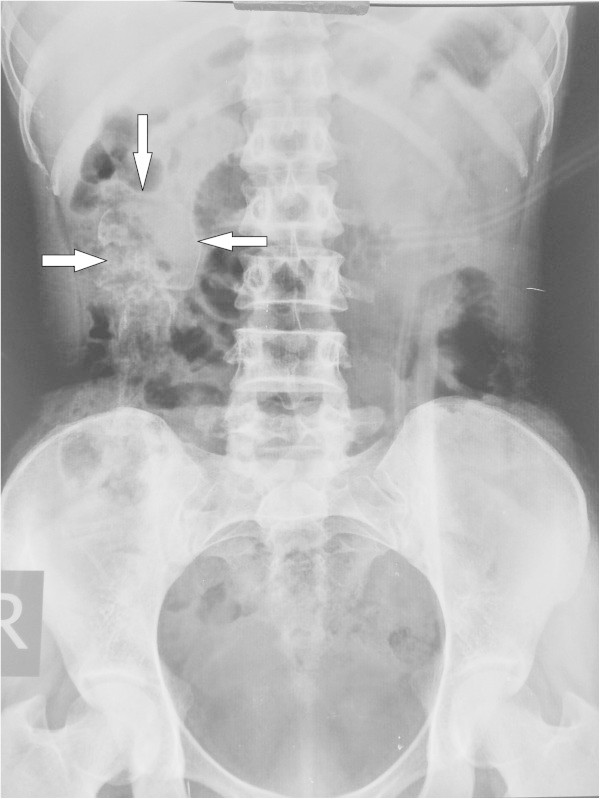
Abdominal radiograph showing radio-opaque shadow of calcified skull of lithopedion.
